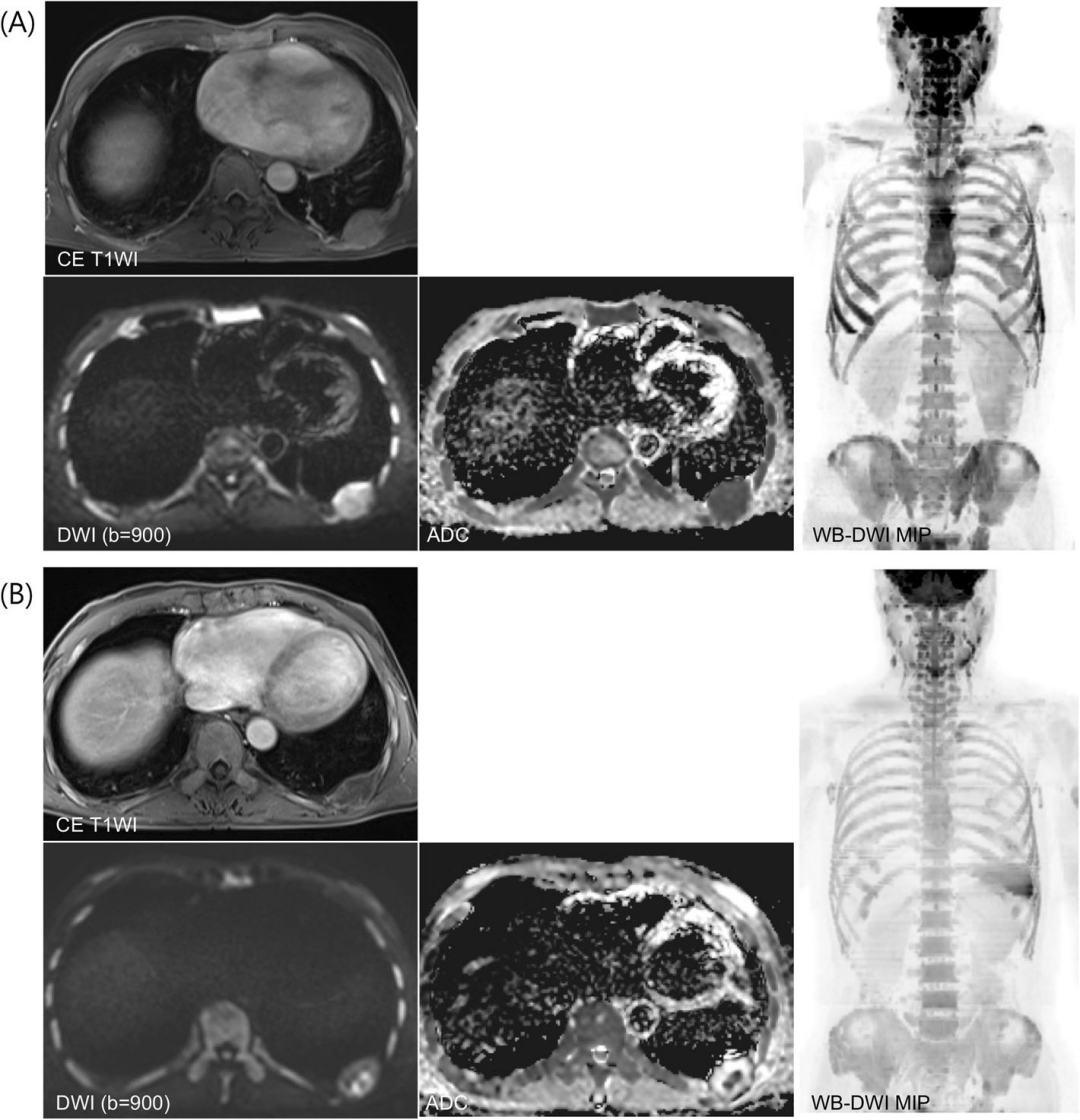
A 56-year-old male with multiple myeloma demonstrating additional benefit of DWI in the evaluation of clinical objective response. MRI taken before chemotherapy (a) shows a 4.0 × 3.1 cm enhancing mass at posterior arc of left 9th rib. Axial DWI (b = 900) and ADC map show marked diffusion restriction in the lesion (mean ADC value: 0.54 × 10^− 3^ mm^2^/s). Coronal diffusion MIP image (b = 900) shows diffuse high signal intensity involving whole axial skeletons, suggesting diffuse bone marrow involvement. After 4 cycles of chemotherapy, follow-up MRI (b) shows equivocal change in the tumor size (3.7 × 2.3 cm) in the left 9th rib. Axial DWI (b = 900) and ADC map show marked improvement of diffusion restriction in the lesion (mean ADC value: 1.54 × 10^− 3^ mm^2^/s). Coronal diffusion MIP image (b = 900) demonstrates decreased signal intensity in the axial skeletons, suggesting good response to the treatment. Based on RECIST 1.1 or MDA criteria, this patient is classified as imaging SD. However, when DWI findings are considered in MDA-DWI criteria, this patient is classified into imaging PR
